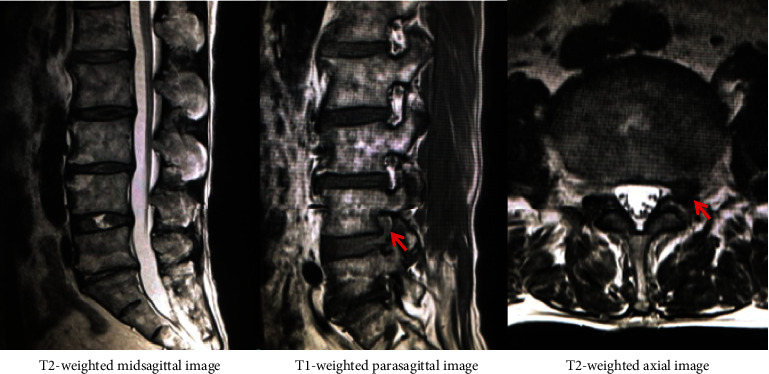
MR images revealed left L4-5 foraminal herniated disc without fragment in the spinal canal.
